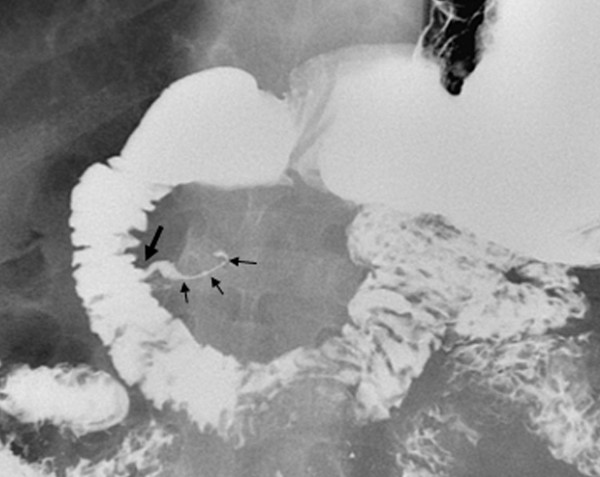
X-ray gastroduodenography with hydrosoluble iodated contrast medium introduced orally showing the diverticulum of the second part of the duodenum. Demonstration of the small neck (large arrow) of the diverticulum. Note that the diverticulum is partially distended by the contrast medium due to the presence of air inside the diverticular lumen (small arrows).
